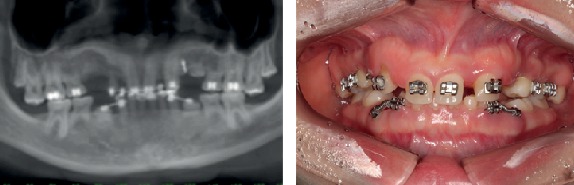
Panoramic radiograph (a) and intraoral buccal view, showing permanent tooth absences and presence of several deciduous teeth.
